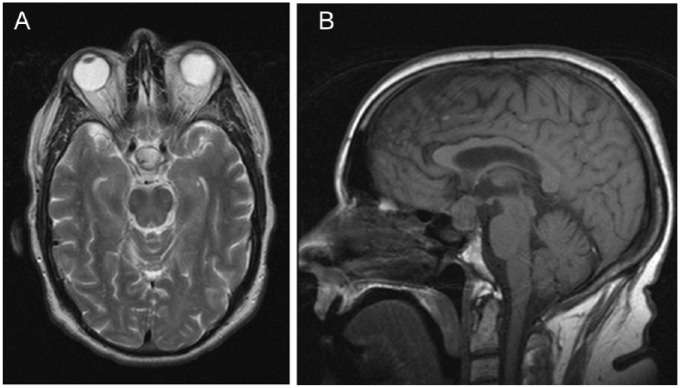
Coronal (A) and sagittal (B) sections of a T2-weighted magnetic resonance image demonstrating a pituitary mass measuring 2.0 × 1.7 × 1.5 cm and extending superiorly with mild displacement of the overlying optic chiasm and optic tracts.
